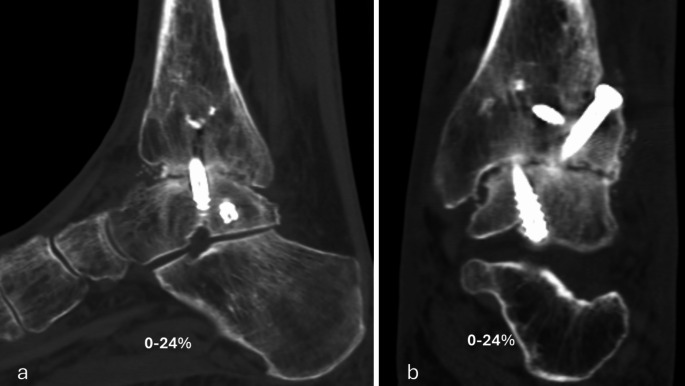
Six months lateral (a) and anteroposterior (b) CT scan of the ankle arthrodesis showing < 25% fusion rate
